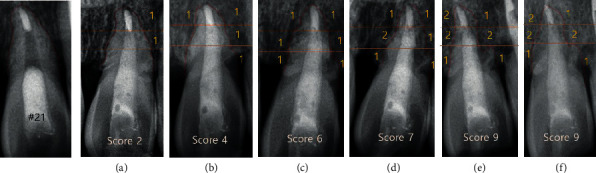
Radiographic index of tooth #21 ((a) 1 year; (b) 2 years; (c) 3 years; (d) 4 years; (e) 4 years: 10 m; (f) 9 years) (Andersson et al.) [14].
